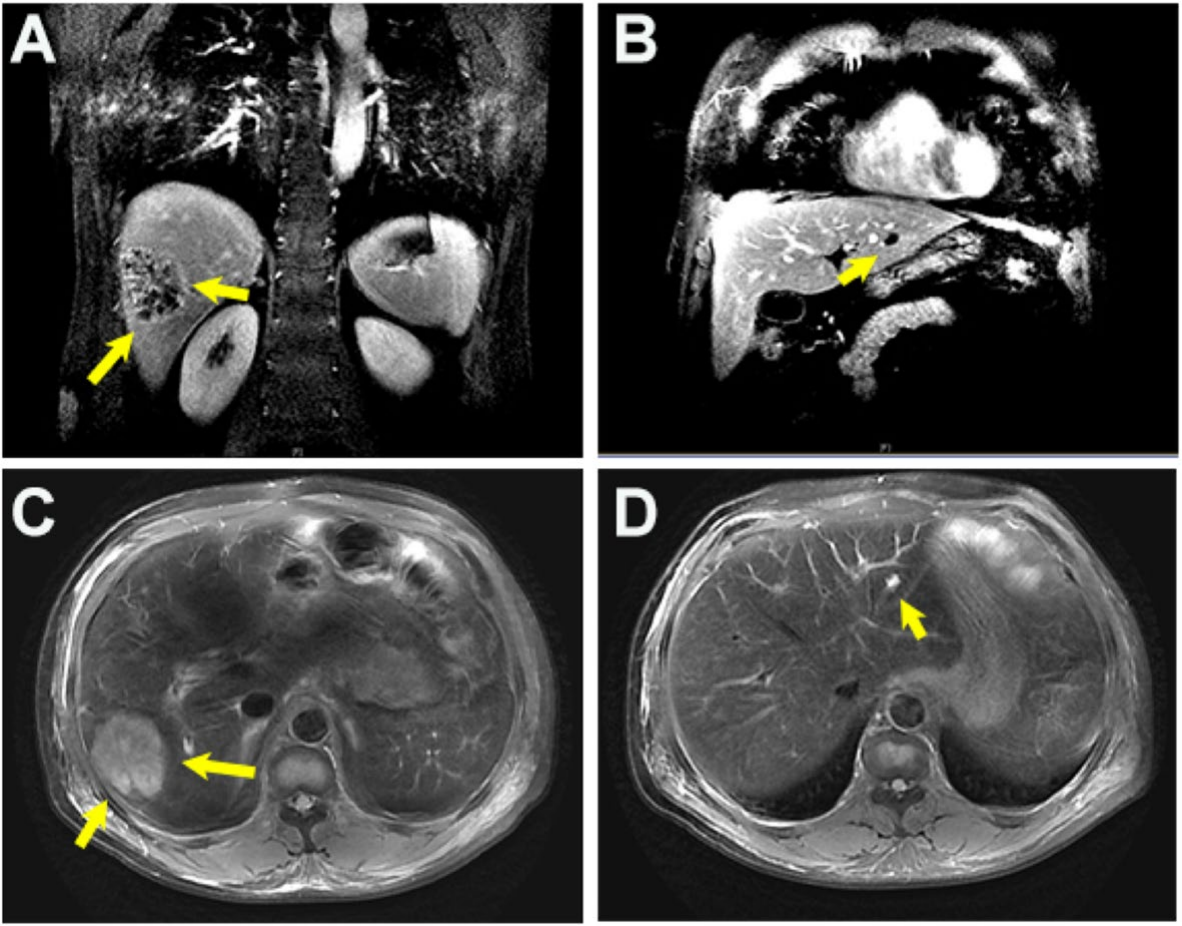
A-D The enhanced magnetic resonance imaging of the liver showed multiple round-like abnormal signals in the liver. After the enhancement, there was obvious uneven ring enhancement. The larger one (*arrow*) was located in the right lobe of the liver and was about 59 × 49 mm in size, suggesting multiple abscesses in the liver
